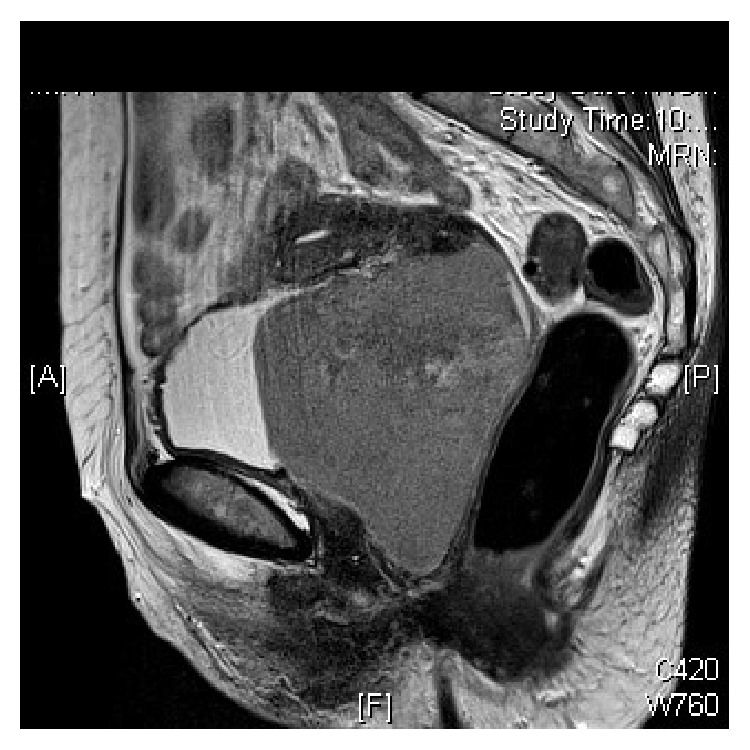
MRI demonstrating the vaginal lesion displacing the uterus towards the cranial direction.
